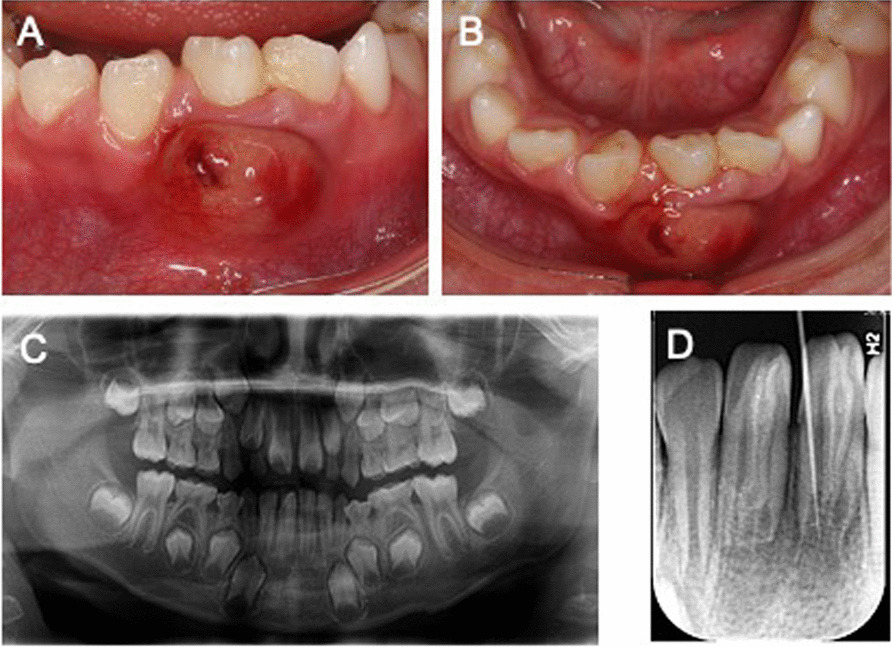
Preoperative clinical view and radiographs of the dens invaginatus (#31and #41): Clinical view shows that the bilateral mandibular central incisors were under eruption with coronal anomaly. (A and B; labial and incisal views, respectively). C The mandibular dental arch panoramic radiograph including the normal number of teeth and anatomical aberration (#31and #41). D Periapical radiograph showing a type III DI extending from the pulp chamber throughout to the apical foramen linked to periapical radiolucency and incomplete root formation
